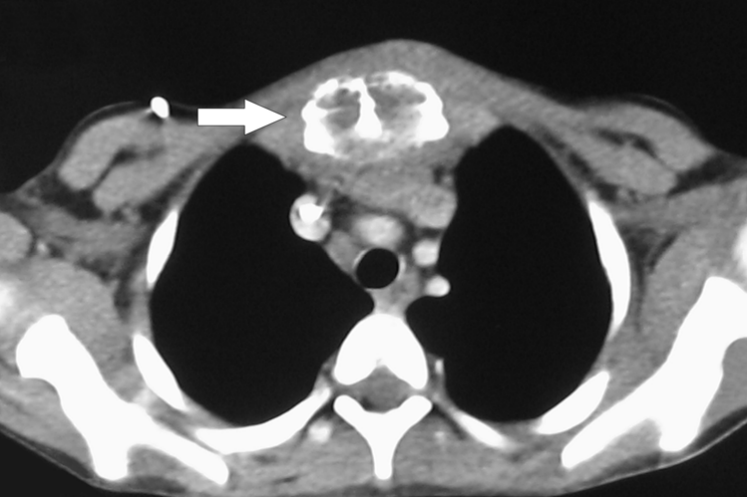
Axial CT of chest. Demonstrating the sternal mass (arrow) but no underlying soft tissue involvement.
